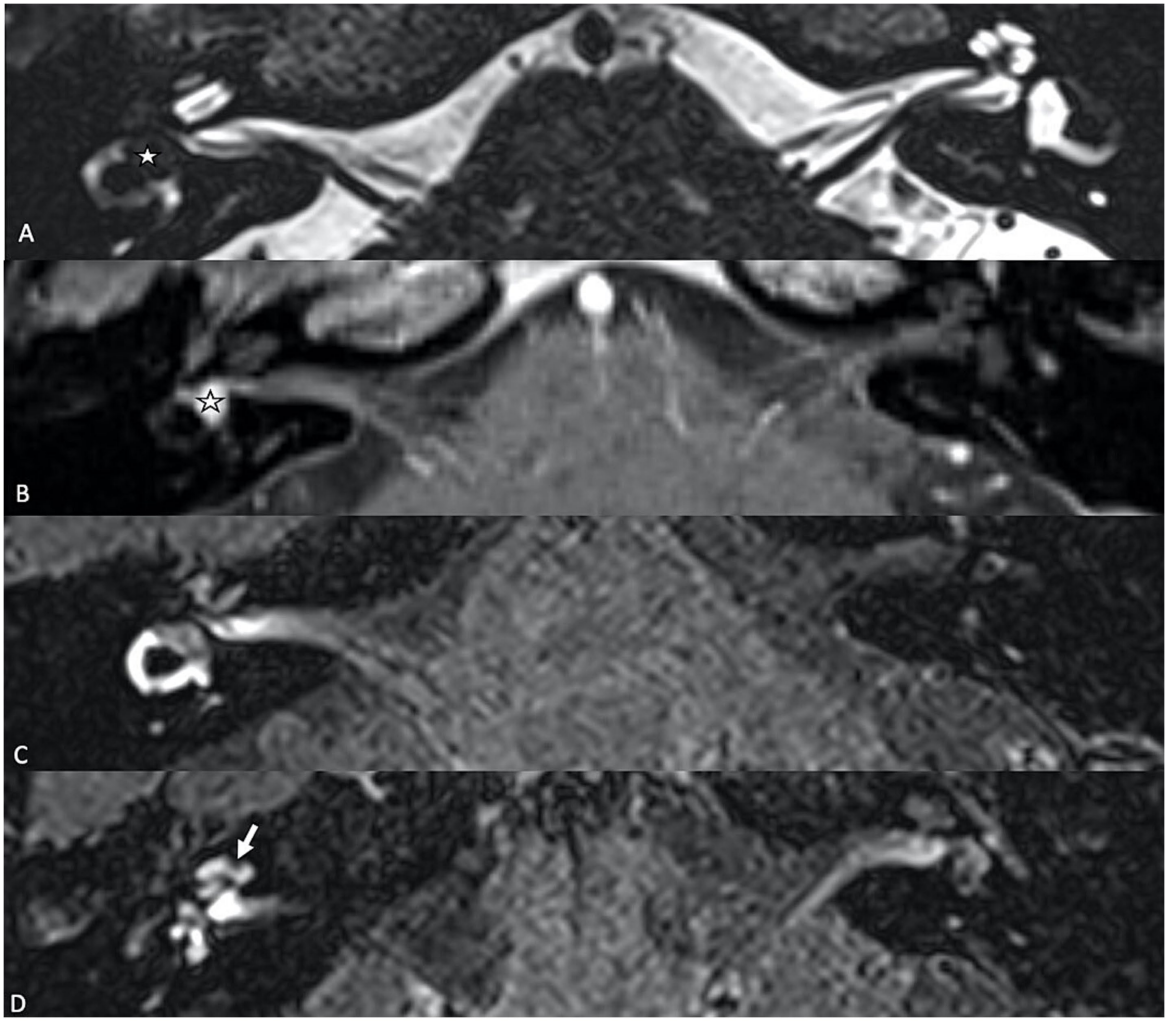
(A) T2-weighted axial MR image showing a primary inner ear (intralabyrinthine) schwannoma (star) in the right vestibular cistern. (B) On the T1-weighted contrast-enhanced image, the tumor appears as a homogenously enhancing nodule (star). (C,D) Delayed 4 h30 FLAIR images showing distension of the right cochlear duct consistent with cochlear endolymphatic hydrops (arrow).
